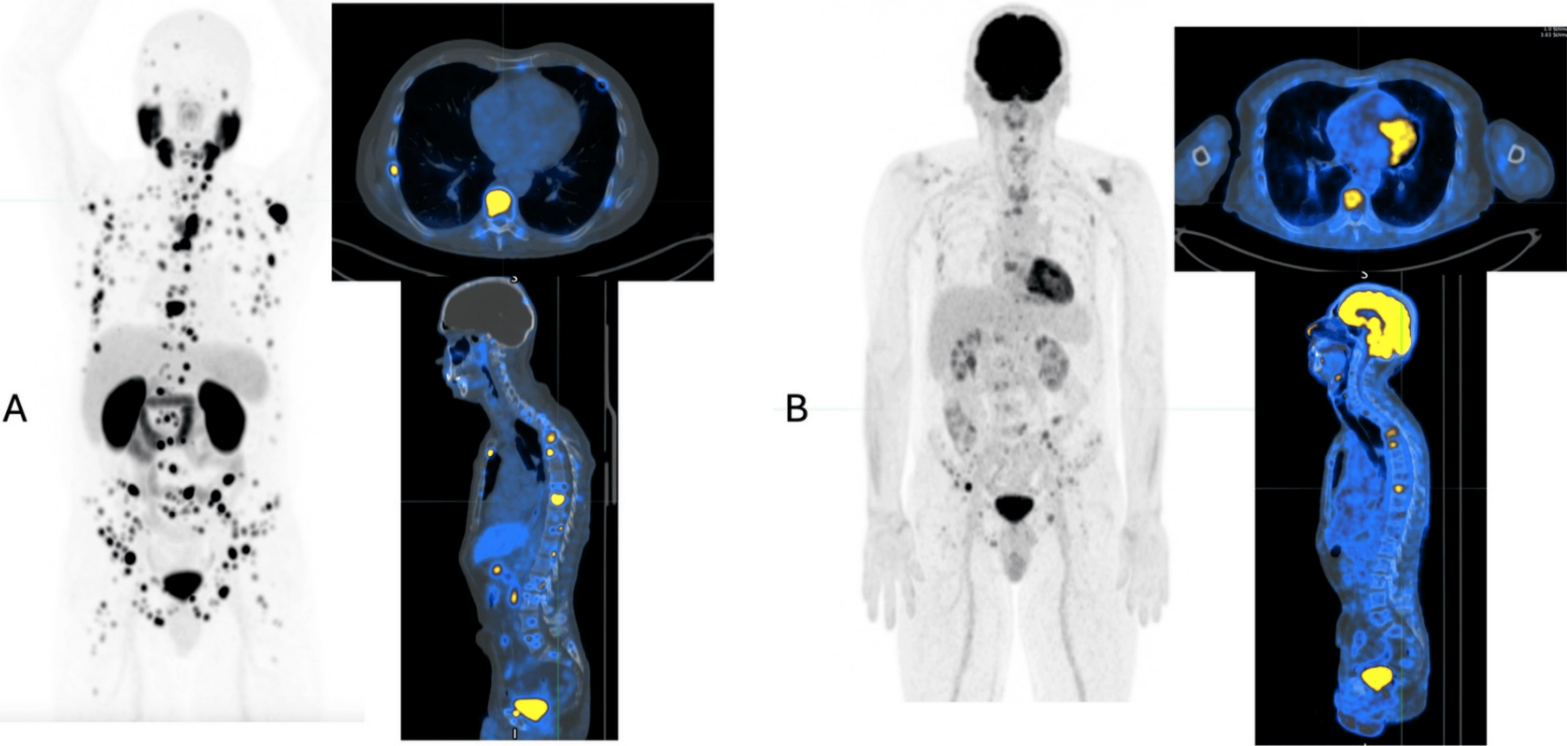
Imaging studies performed at the PET scan unit, Hospital Metropolitano, Quito, Ecuador. (A) ^68^Ga-PSMA PET showing multiple lesions with overexpression of PSMA receptors involving the skeleton and the prostate bed, according to the standardized molecular imaging classification system PROMISE v2 (Prostate Molecular Imaging Standardized Evaluation): mITr, N0, M1b diss [4]. (B) ^18^F-FDG PET demonstrating hypermetabolic osseous lesions disseminated throughout the axial and appendicular skeleton. ^18^F-FDG: fluorine-18 fluorodeoxyglucose; ^68^Ga-PSMA: gallium-68 prostate-specific membrane antigen
